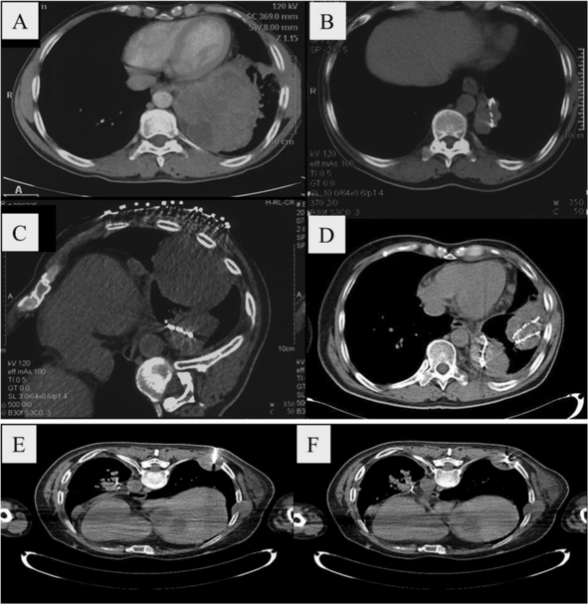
CT images of a squamous cell lung carcinoma in the lower left lung in a 56-year-old man (No. 3) with brain metastasis: (a) before treatment; (b) 12 months after the first iodine-125 seed implantation of iodine-125 seeds; (c) 30 months after the first implantation showing a recurrent tumor mass (8 × 4 cm^2^) in the lower left thoracic cavity; (d) 33 months after the first, i.e. 3 months after the second implantation, showing that the tumor size had decreased by 50 %; (e) during the third implantation, i.e., 42 months after the first implantation, showing metastasis on the right side of the chest wall; (f) Immediately after the third implantation
